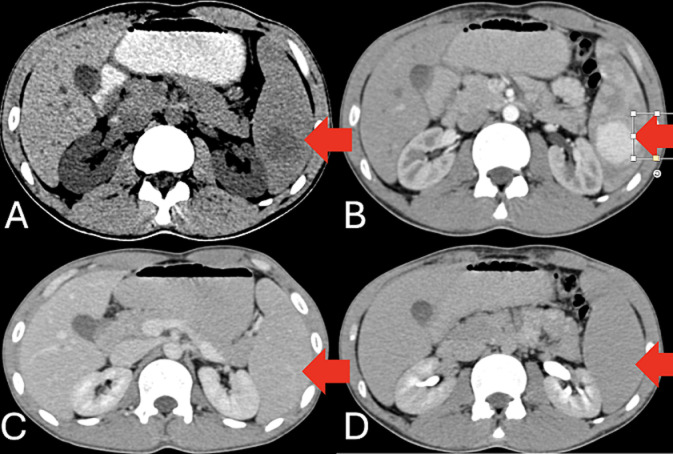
contrast-enhanced computed tomography image of abdomen in axial section showing hyperdense (A); enhancing lesion noted at lower pole of spleen which is enhancing in arterial (B); phase with rapid washout on portal (C) and delayed phase (D) suggestive of splenic hamartoma
